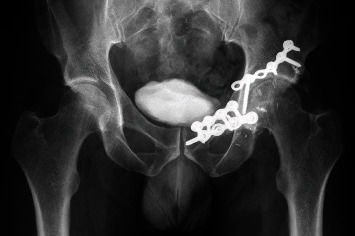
Plain film at 30 months of follow-up showing a mild thinning of the joint line.
